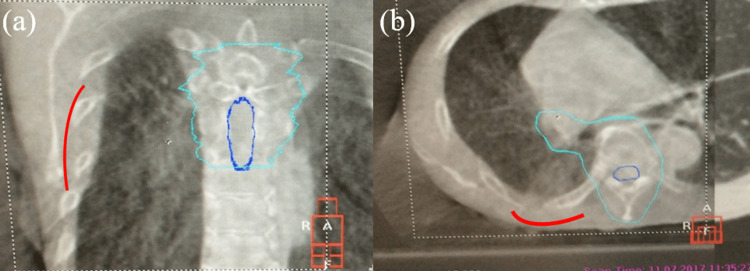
CBCT images displayed in (a) coronal and (b) axial planes RP was suggested by the red lines. Sky-blue and blue lines show the PTV and spinal cord contours, respectively. RP - radiation pneumonitis; PTV - planning target volume
